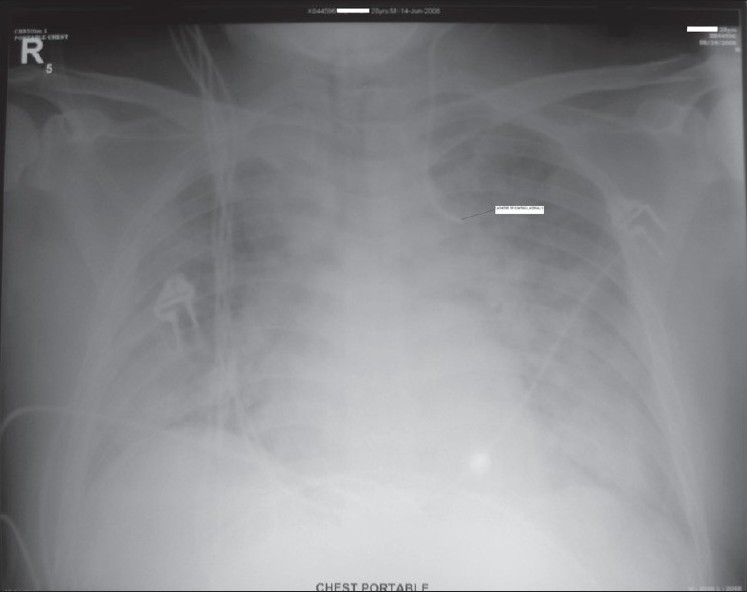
Anteroposterior Chest Radiograph showing central venous catheter lying in the left paramedian location following the aortic knob
